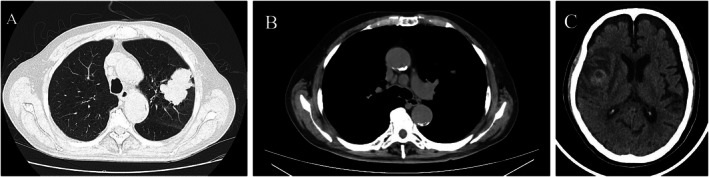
Radiologic findings of primary pulmonary leiomyosarcoma. (A) Axial chest computed tomography (CT) image obtained prior to EBUS‐TBNA showing a solitary mass in the left upper lobe. (B) Axial CT image obtained prior to EBUS‐TBNA showing an enlargement of the 4L lymph node. (C) Non‐contrast brain CT image showing a solitary low‐density lesion with surrounding edema in the right cerebral hemisphere, consistent with brain metastasis.
